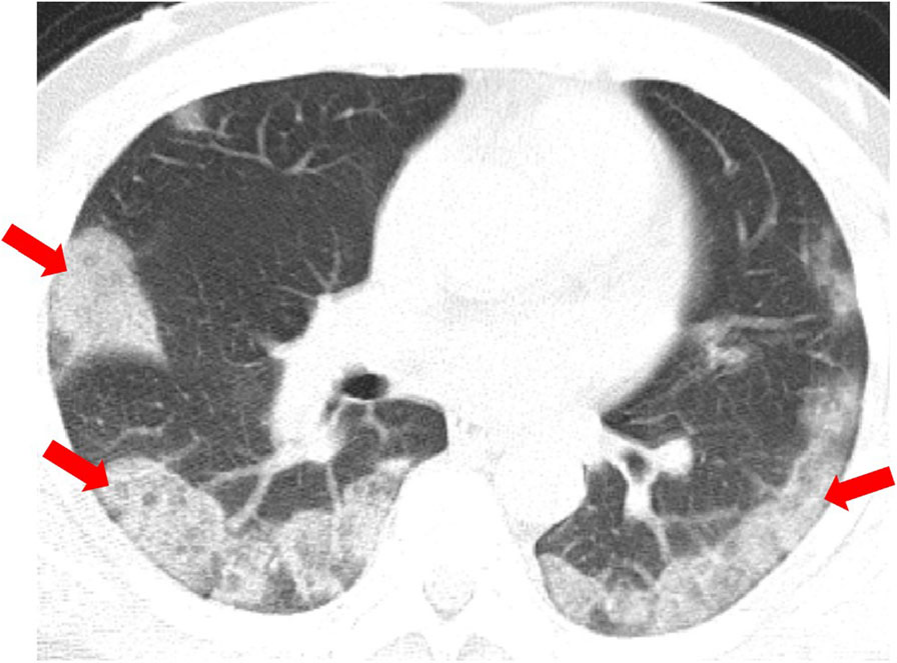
A male patient infected with SARS-CoV-2 who presented severe difficulty in breathing. Transverse CT performed on six days after onset of symptoms shows peripheral ground glass opacities (red arrow) under the pleura of the middle and lower lobes in both lungs
